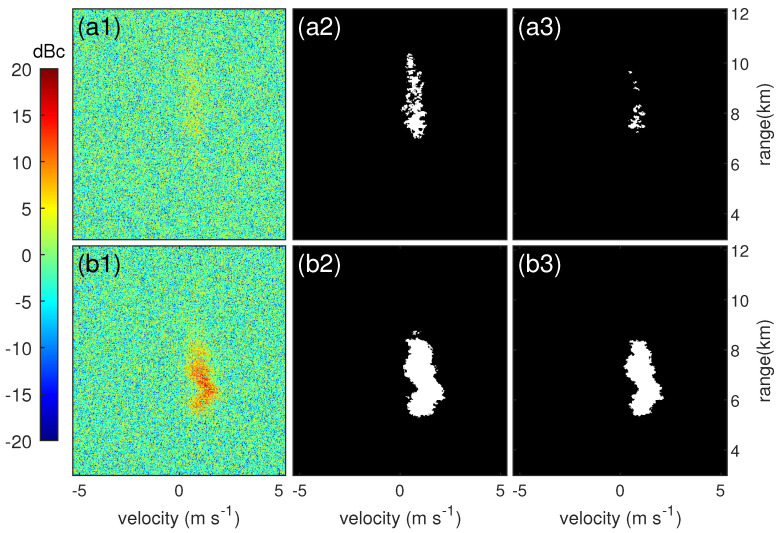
Impact of noise level estimation deviation on weak cloud signals. (a1) and (b1) are the raw Doppler power spectrum. (a2) and (b2) are cloud signal mask results with noise levels estimated manually. (a3) and (b3) are cloud signal mask results with noise levels 1 dB higher than (a2) and (b2).
